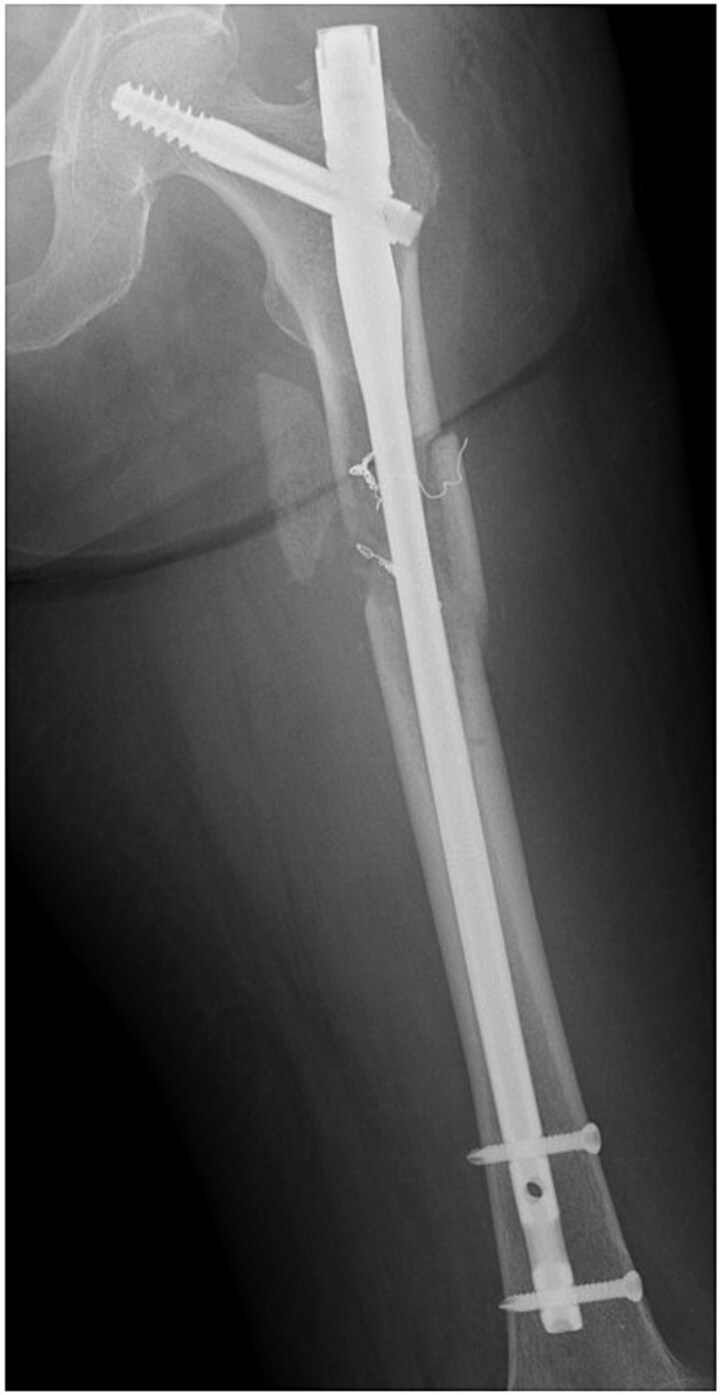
Postoperative radiograph of the left femur.
